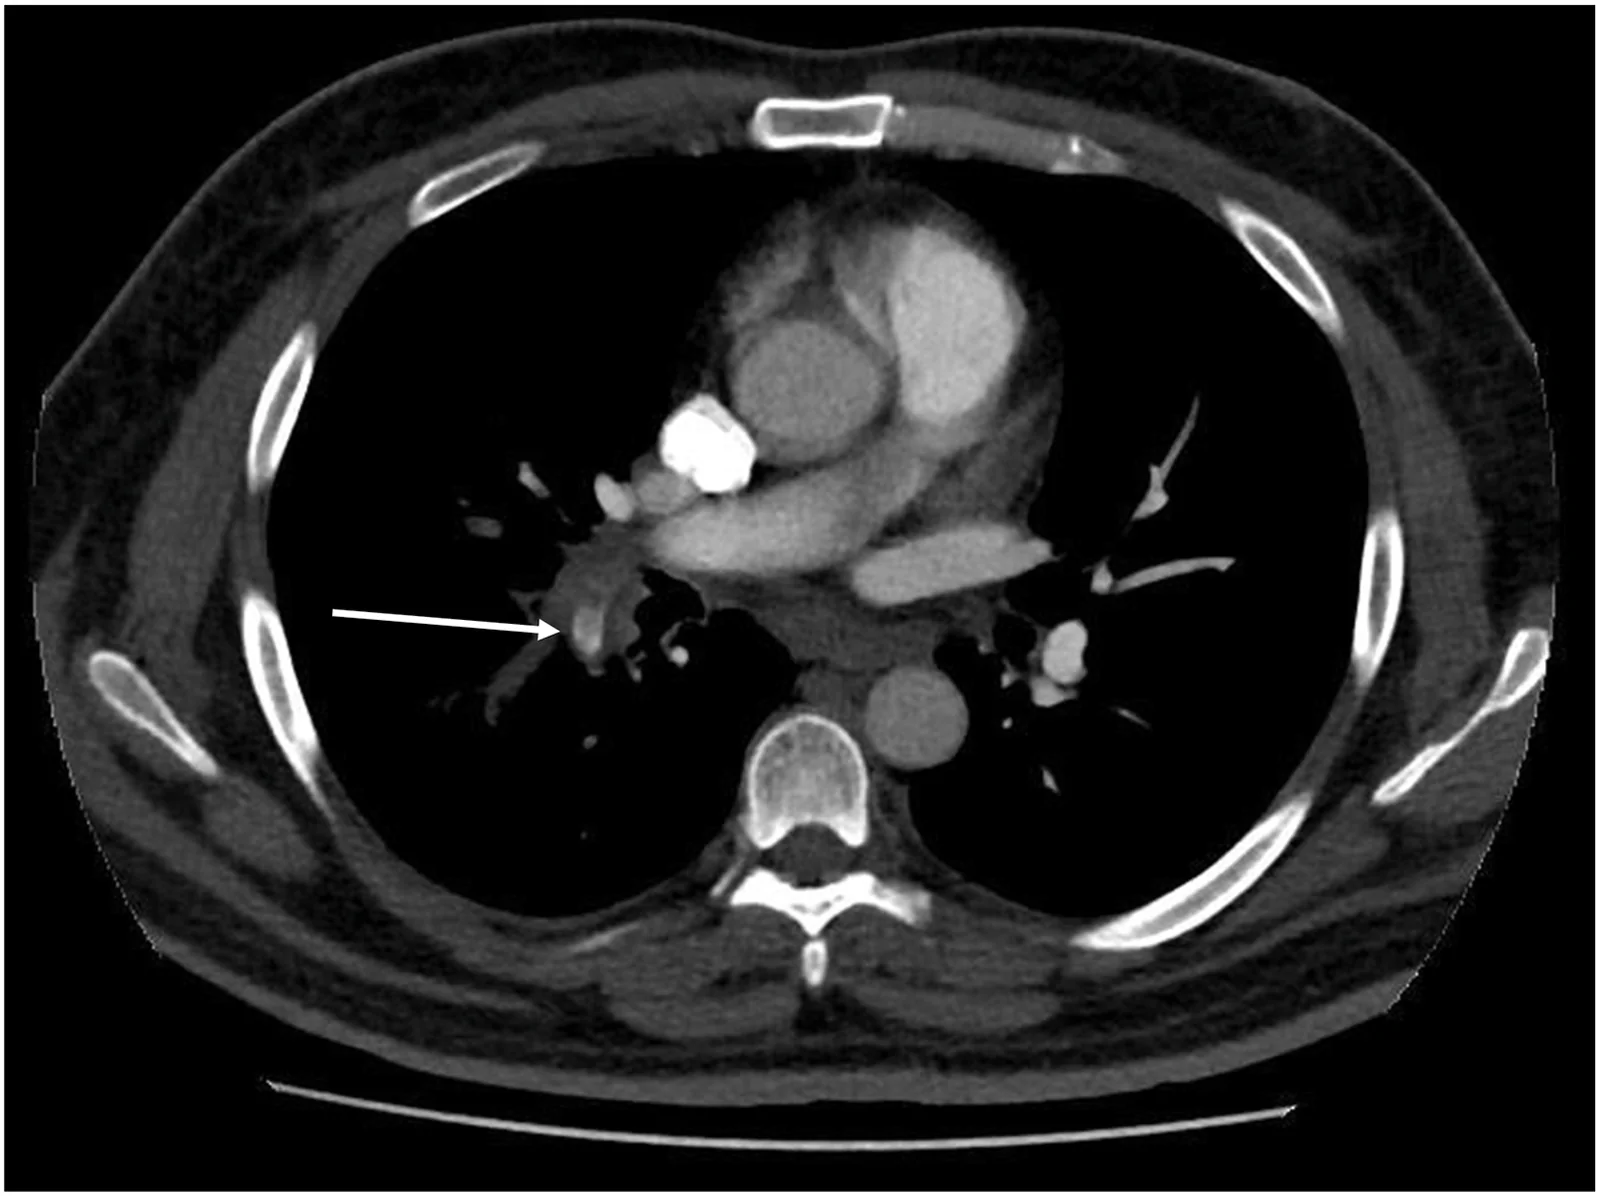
Computed tomography pulmonary angiogram (axial view) demonstrates segmental pulmonary arterial filling defects consistent with septic pulmonary emboli (*arrow*, filling defect in right pulmonary artery branch).
